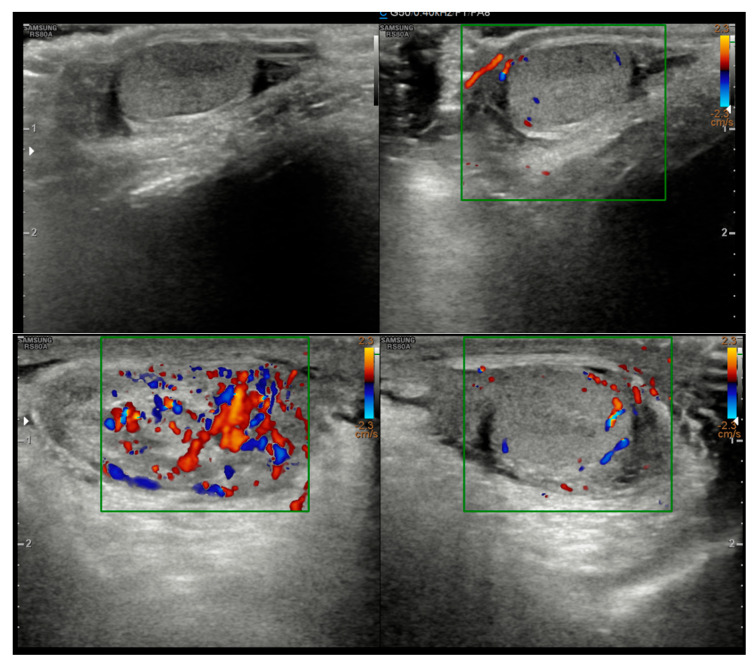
Initial ultrasound showing a thickened left spermatic cord with increased vascular signals.
